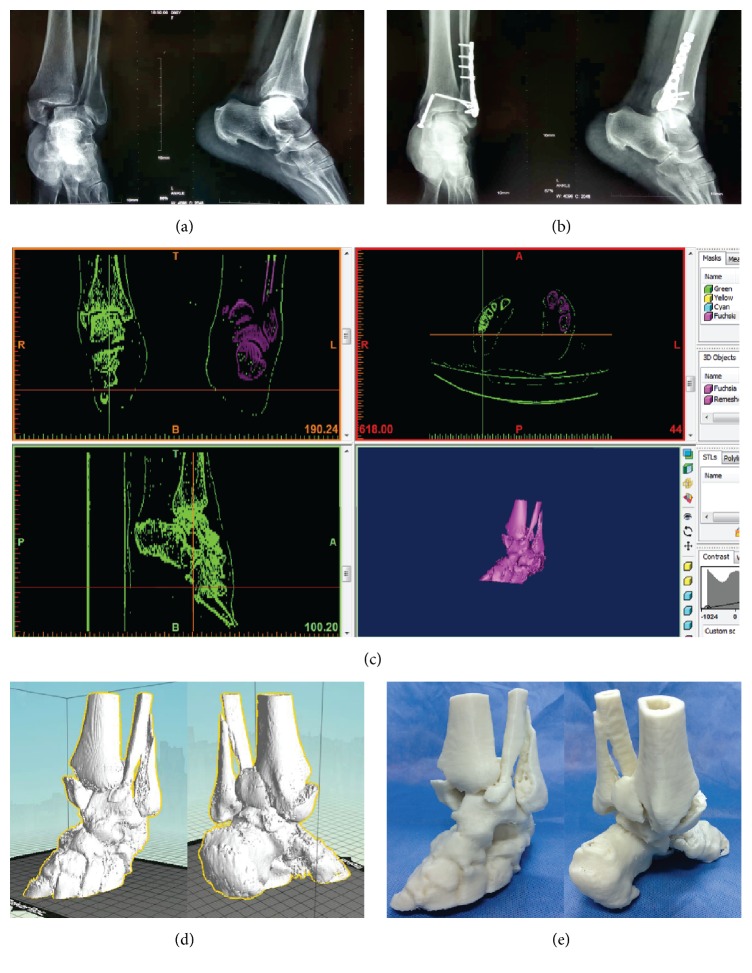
A 37-year-old female had a left trimalleolar fracture and underwent open reduction and internal fixation. 3D prototype was designed and printed by the authors; it was used for preoperative planning: (a) preoperative X-ray film; (b) postoperative X-ray film; (c) preoperative three-dimensional reconstruction by Mimics software; (d, e) digital fracture prototype and the corresponding 3D-printed 1 : 1 solid prototype.
